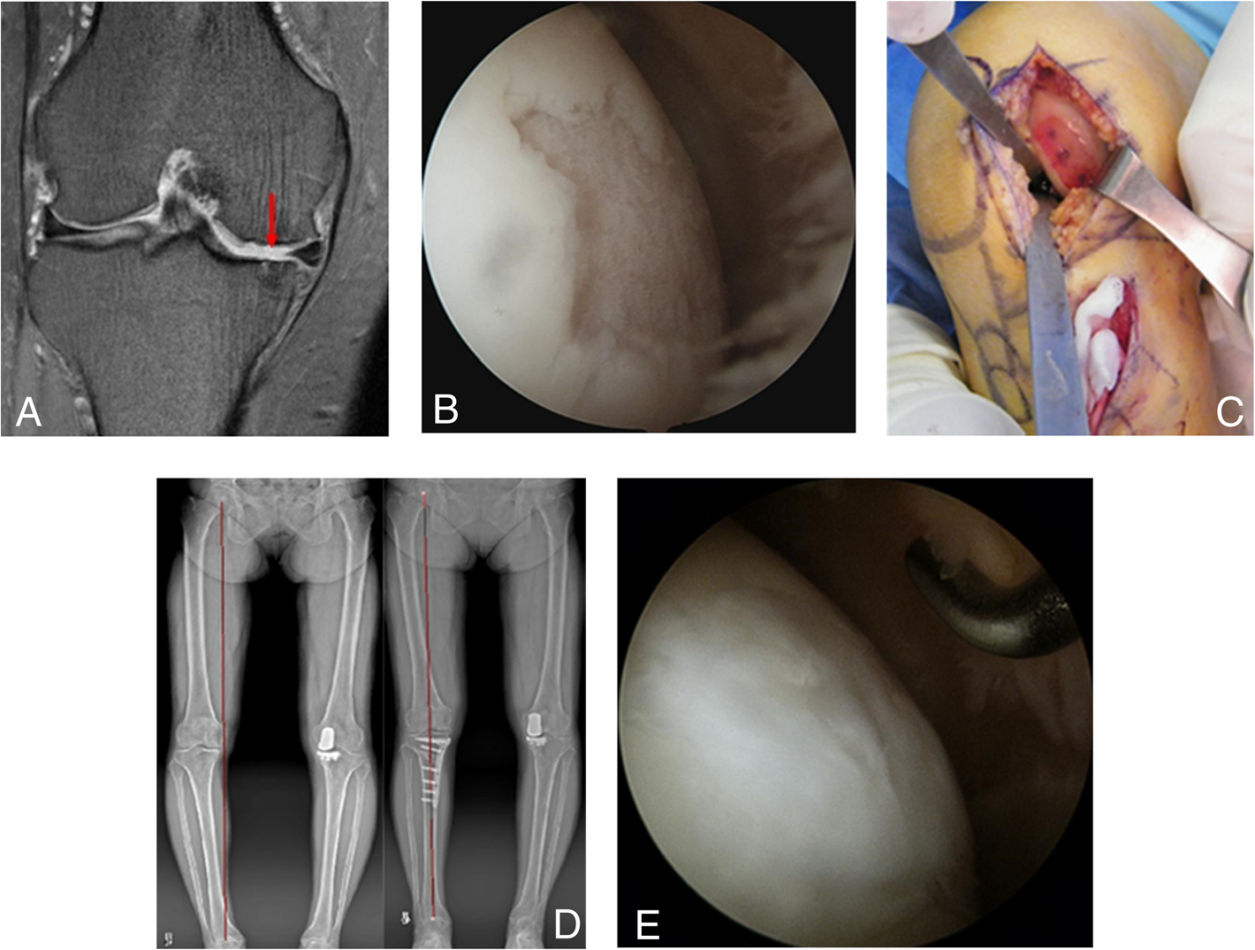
This case was a 59-year-old female suffering from medial osteoarthritis with right knee pain. a Large cartilage defect on medial femoral condyle through preoperative MRI. b 3.0-cm^2^-sized cartilage defect was confirmed by diagnostic arthroscopy. c Concomitant ACI was performed. d The mechanical axis could be corrected from 10° varus preoperatively to neutral alignment at the final follow-up. e The cartilaginous regeneration (ICRS score, 10 points) could be confirmed at the second-look diagnostic arthroscopy
